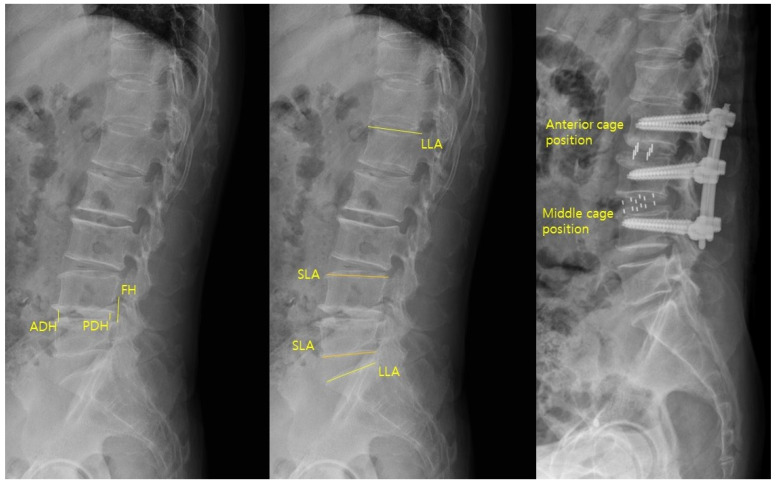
Sagittal parameters of ADH, PDH, FH, SLA, and LLA were measured on lateral radiographs, and cage position was categorized as anterior or middle based on its location on the inferior endplate.
